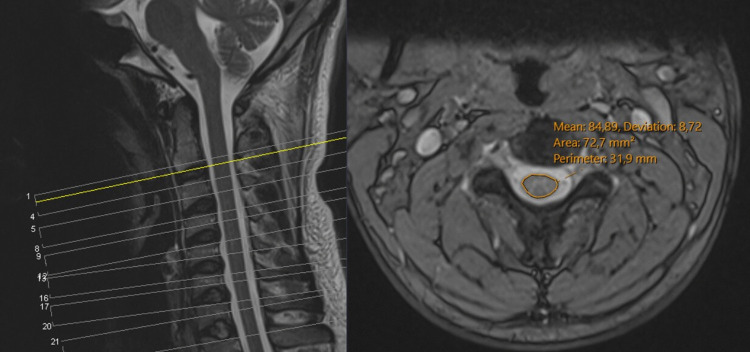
Measurement of the cervical spinal cord cross-sectional area at the C2–C3 level using axial T2-weighted MRI
